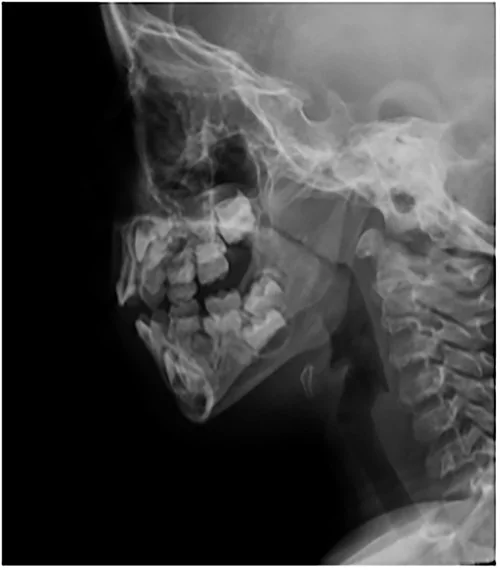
Preoperative lateral soft tissue neck X-ray showing enlarged adenoidal tissue nearly obstructing the nasopharynx.
